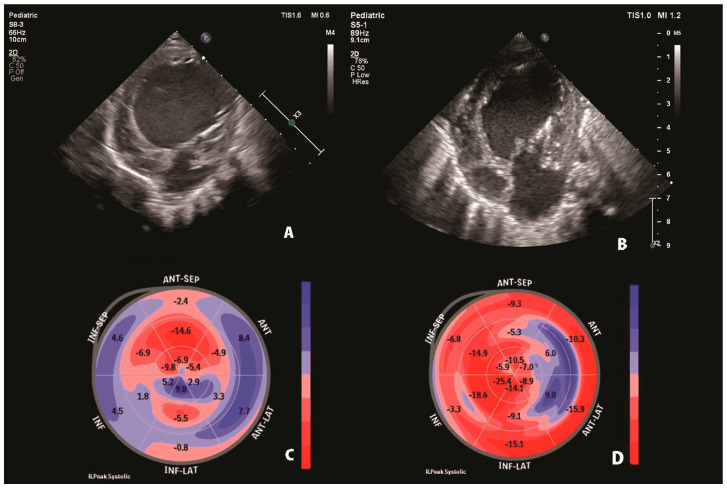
Compared to preoperative echocardiography, follow-up 2D echocardiography showed a mildly dilated left ventricle, with reverse remodelling after surgery (A,B). Follow-up speckle tracking analysis revealed that postoperative global longitudinal strain increased compared to preoperative analysis, with normal global longitudinal strain in right coronary artery territories. At the same time, mechanical dyssynchrony remained in some segments supplied by the left coronary artery (C,D).
